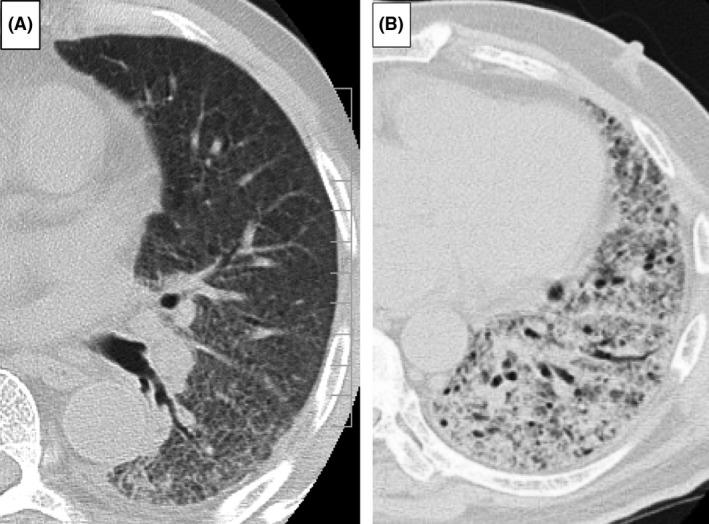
Representative chest high‐resolution computed tomography (CT) images of patients with acute respiratory distress syndrome lacking common risk factors who were treated with high‐dose steroid therapy. A, Chest high‐resolution CT in an 81‐year‐old man alive 60 days after initiation of therapy (survivor) showing ground‐glass attenuation predominance. B, Chest high‐resolution CT in a 76‐year‐old male non‐survivor showing consolidation predominance with bronchial dilatation.
